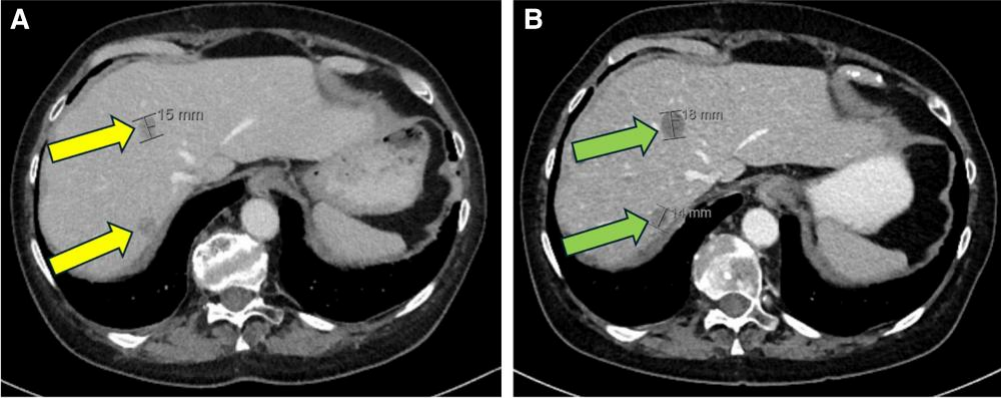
CT of the abdomen with IV contrast at 8 months (A) and at 11 months (B) after surgery revealing new hepatic lesions consistent with metastatic ACC (yellow arrows) increasing in size at 3 months interval (green arrows).
